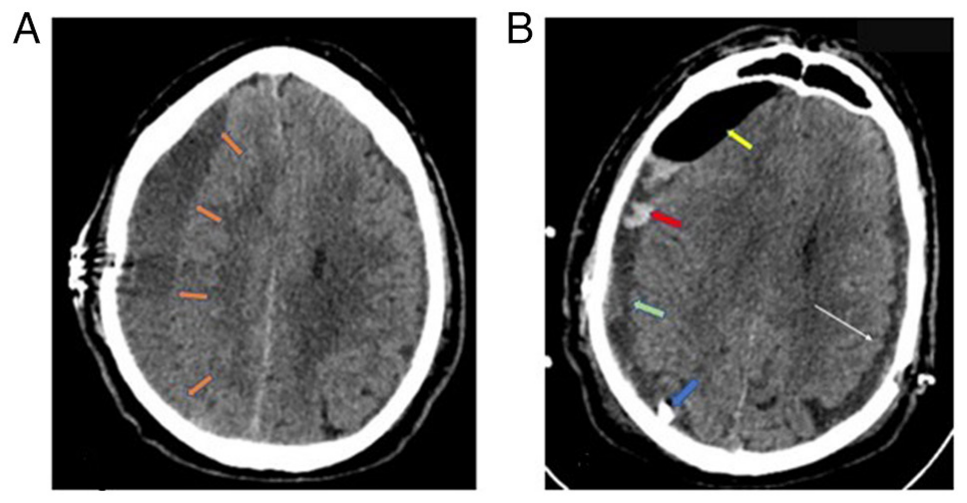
(A) At 2 weeks after the initial hematoma evacuation, the patient returned with recurrence of the right-side hematoma (orange arrows). (B) The post-operative computed tomography scan revealed partial hematoma evacuation (green arrow) with acute hemorrhage on a top of chronic subdural hematoma (red arrow) and air (yellow arrow) in the subdural space. The blue arrow indicates the draining catheter, whereas the white arrow points to the recurrence of the left-side hematoma.
